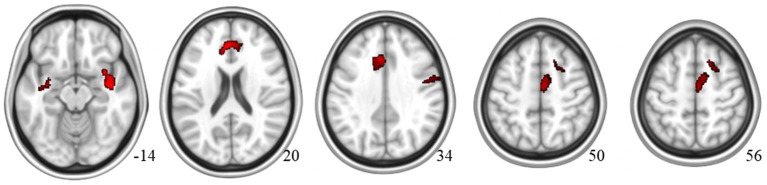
Brain regions showing significant GM volume changes associated with THI score in MRI.
